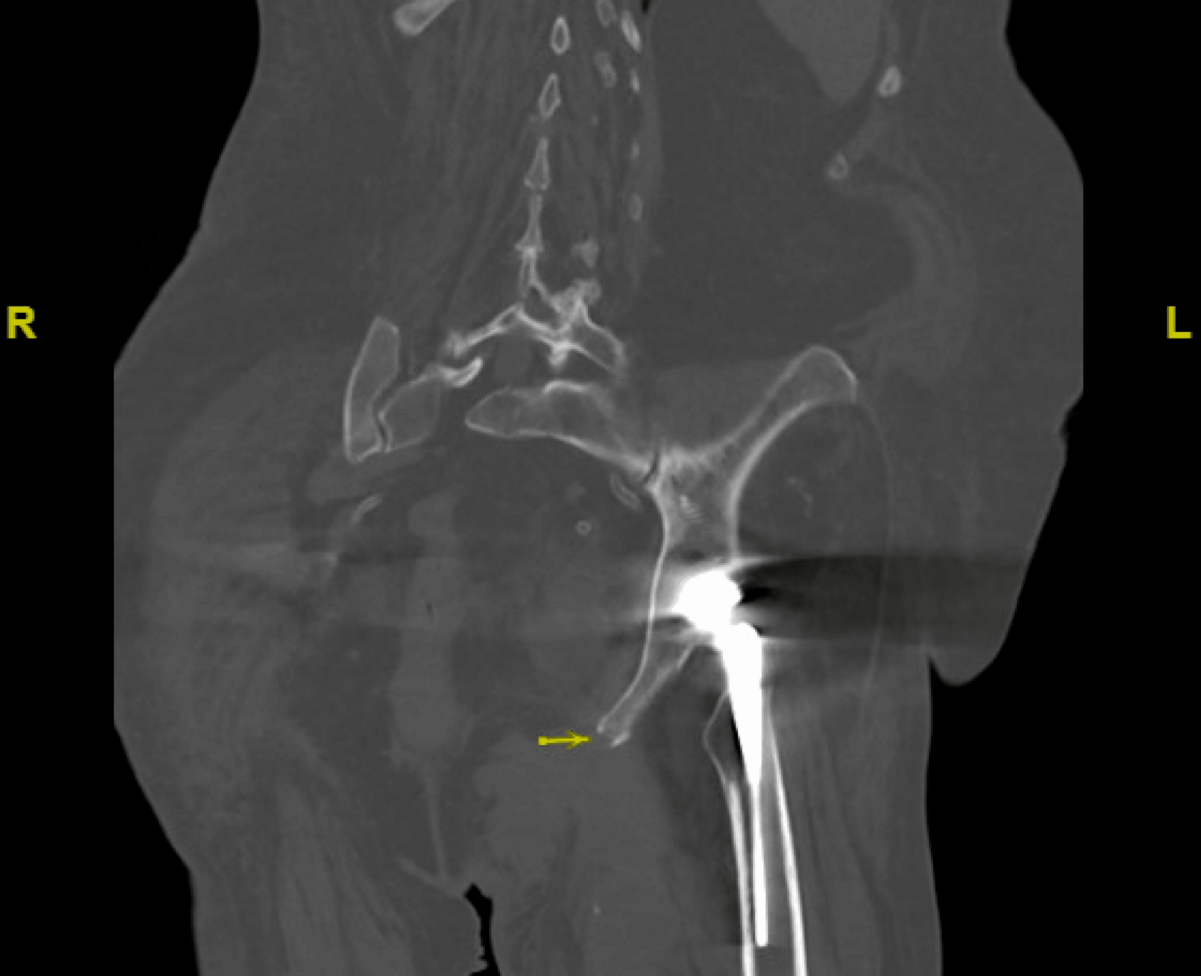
Coronal non-contrast CT of the abdomen and pelvis Series 4, images #99–106, showing cortical loss of the posterior left inferior pubic ramus with extension into the ischial tuberosity (yellow arrow), which was concerning for osteomyelitis.
